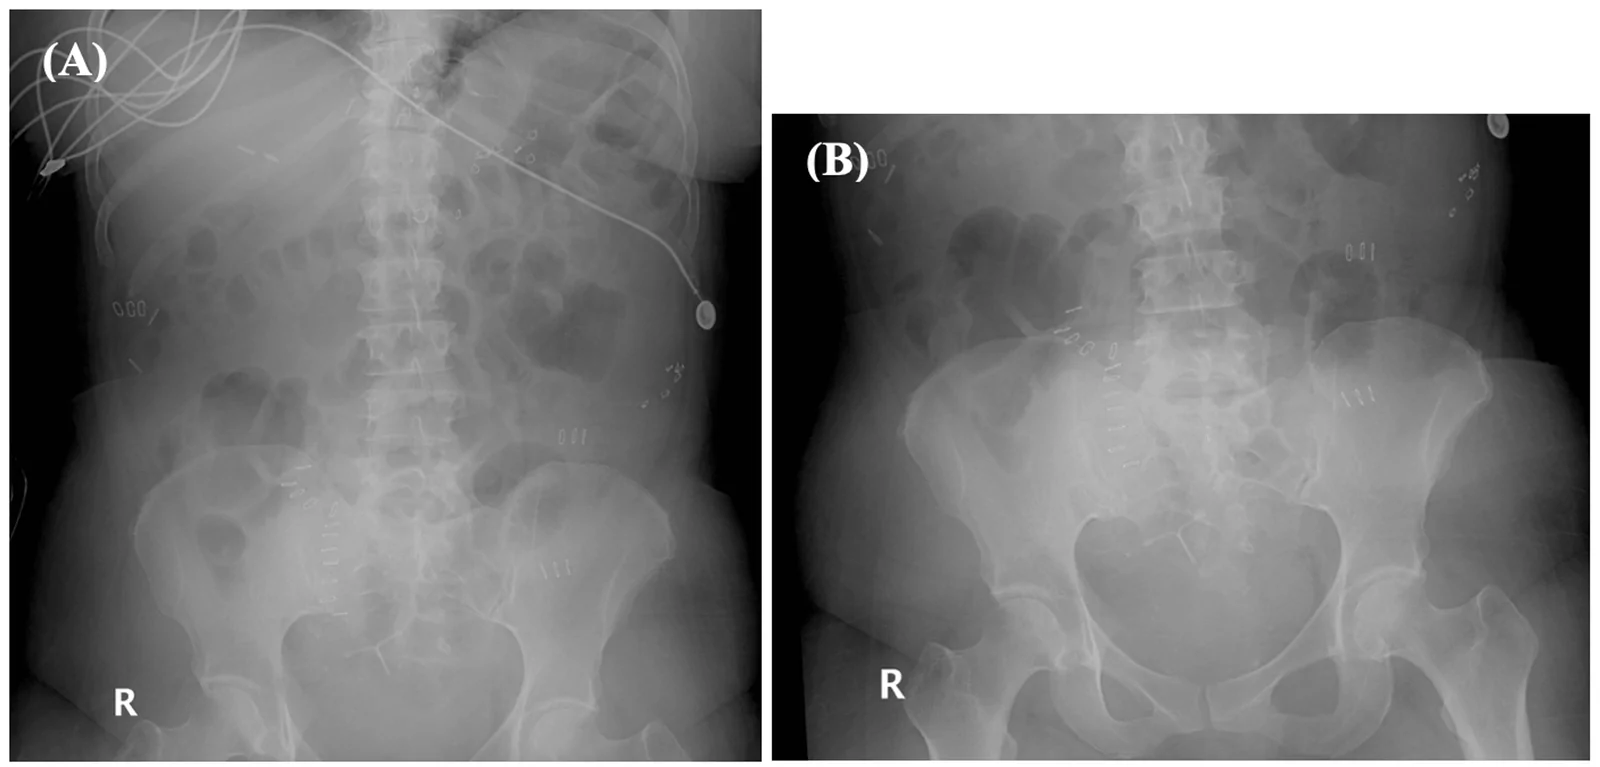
Preoperative abdominal radiographs with nonspecific findings for small bowel obstruction. (A) Supine abdominal radiograph showing nonspecific bowel gas distribution with a paucity of gas in the left colon and pelvis and no definitive radiographic evidence of small bowel obstruction.
